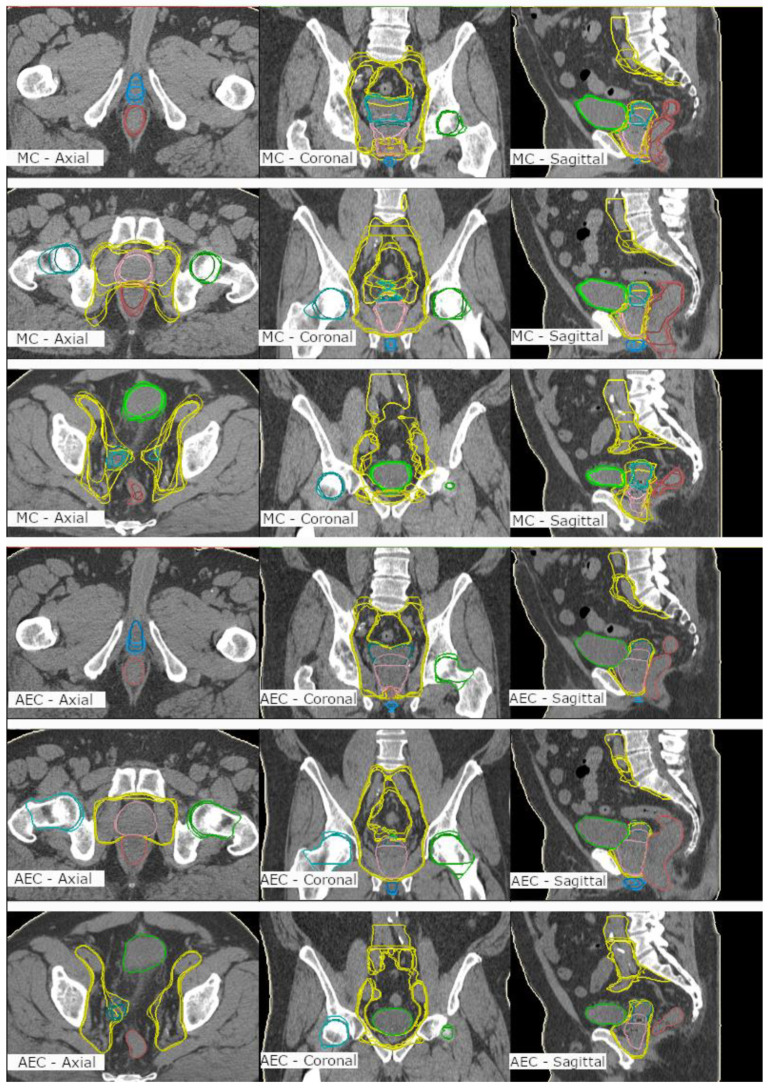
Manual contouring (MC) (upper 9 images) and automated and edited contouring (AEC) (lower 9 images) of prostate (pink), seminal vesicles (turquoise), lymph nodes (yellow), bladder (light green), rectum (brown), femoral heads (green&light turquoise) and penile bulb (blue) by four oncologists on one patient’s computed tomography scan. Left column: axial plane, middle column: coronal plane; right column: sagittal plane.
